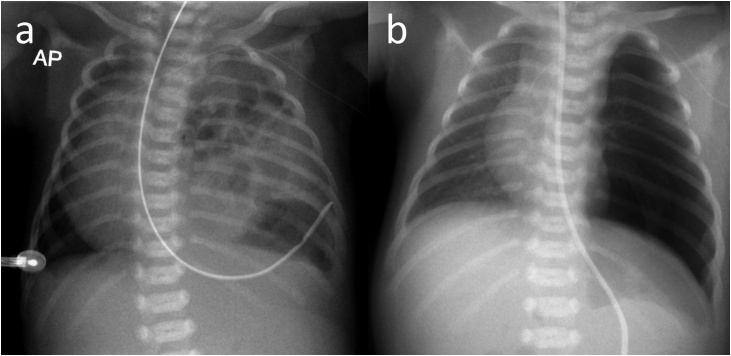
A) Preoperative image: Chest X-ray showed intestinal herniation into the thoracic cavity. B) Postoperative image.
